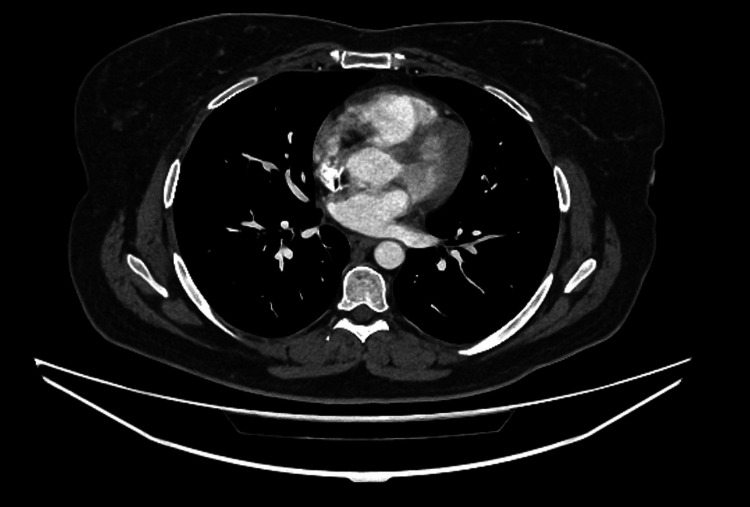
Computed tomography pulmonary angiography (CTPA) sagittal view.
